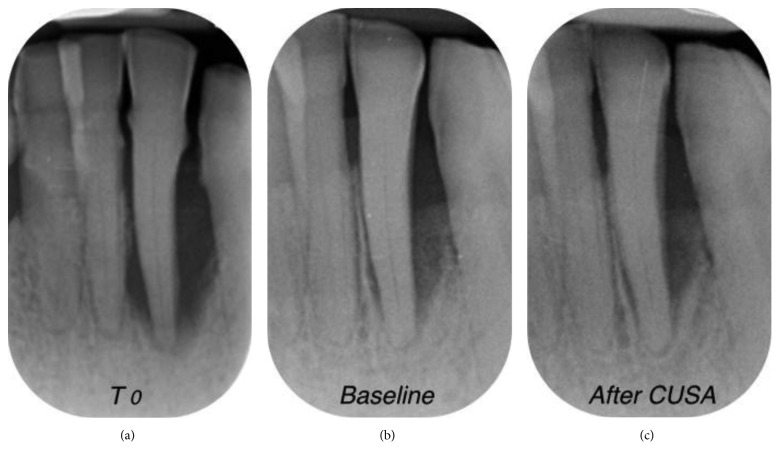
Progression of X-rays showing bone remineralization. Baseline is 12 months after (a) T0. In this case, the PD decreased from 10 mm ((b) baseline) to 4 mm (6 months (c) after CUSA). The CAL gain was 6 mm (from 14 mm to 8 mm).
